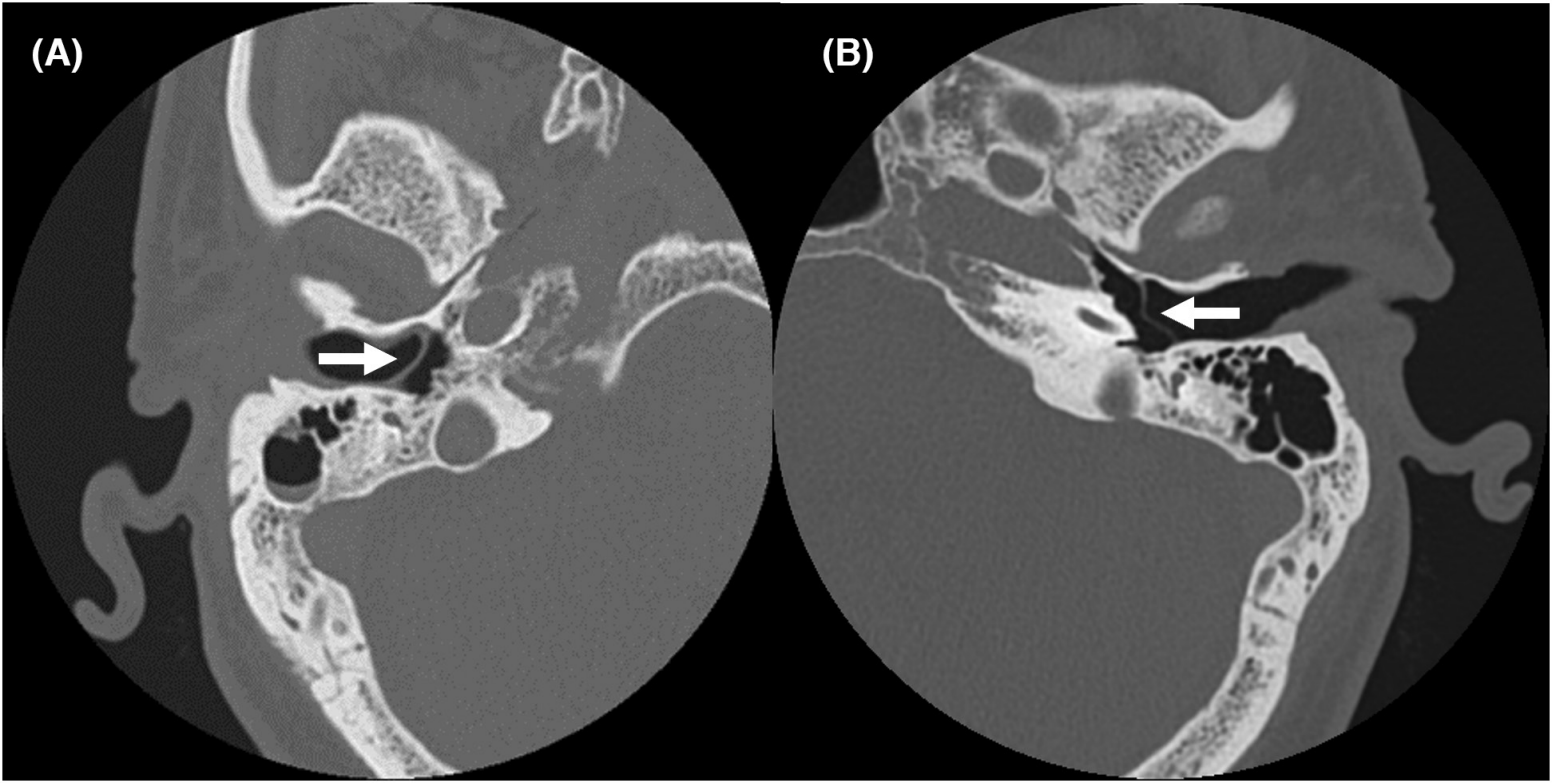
Temporal bone axial CT showing right TM (arrows) thickened with narrowed EAC (A) compared to the normal left TM and EAC (B).
